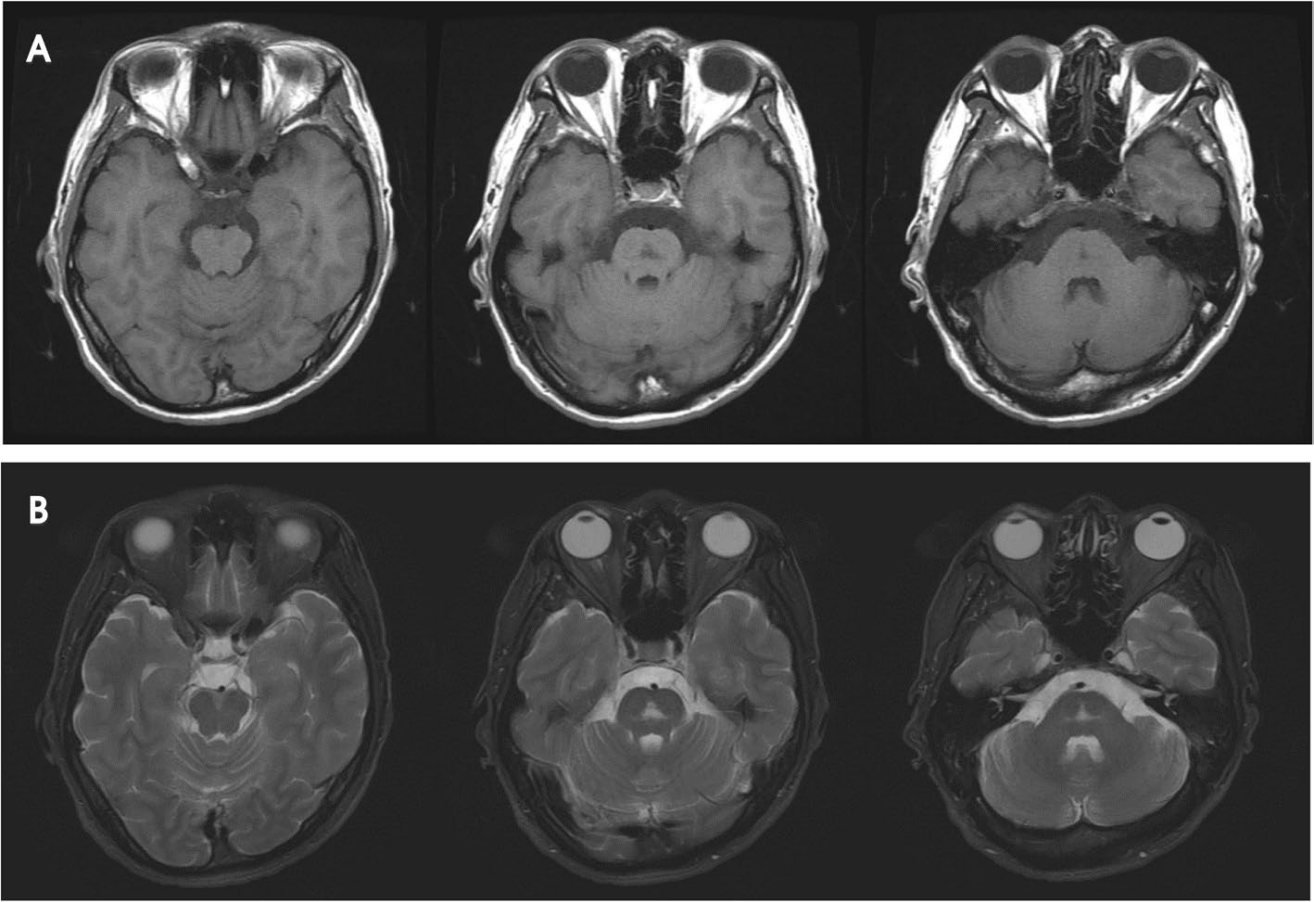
Brain MRI at 10 months after taking tacrolimus shows a low signal intensity lesion in the middle of the pontine tegmentum on a T1 weighted image (A) and high signal intensity lesion in the same region on a T2 weighted image (B)
